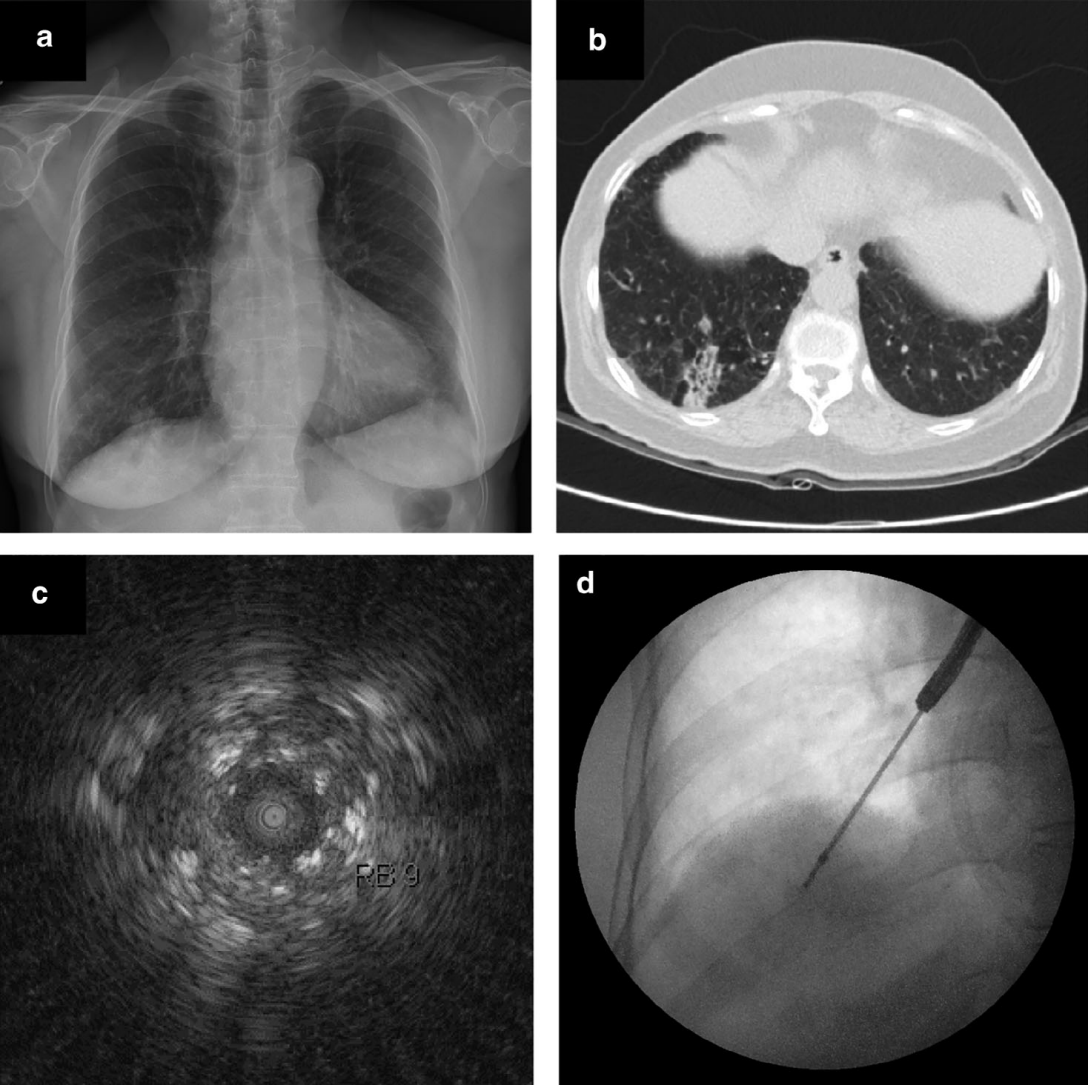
Case of a 74‐year‐old man with a consolidation in the right lower lobe.(a) Chest X‐ray showing consolidation at the right lower lobe. (b) Computed tomography (CT) scan showing a 38 mm peripheral lung mass. (c) Radial probe endobronchial ultrasound (R‐EBUS) in the lateral basilar segment of the right lower lobe revealing a mixed blizzard sign containing a heterogeneous acoustic shadow with hyperechoic dots, linear arcs, and vessels. (d) Fluoroscopic image during transbronchial biopsy through a guide sheath.
